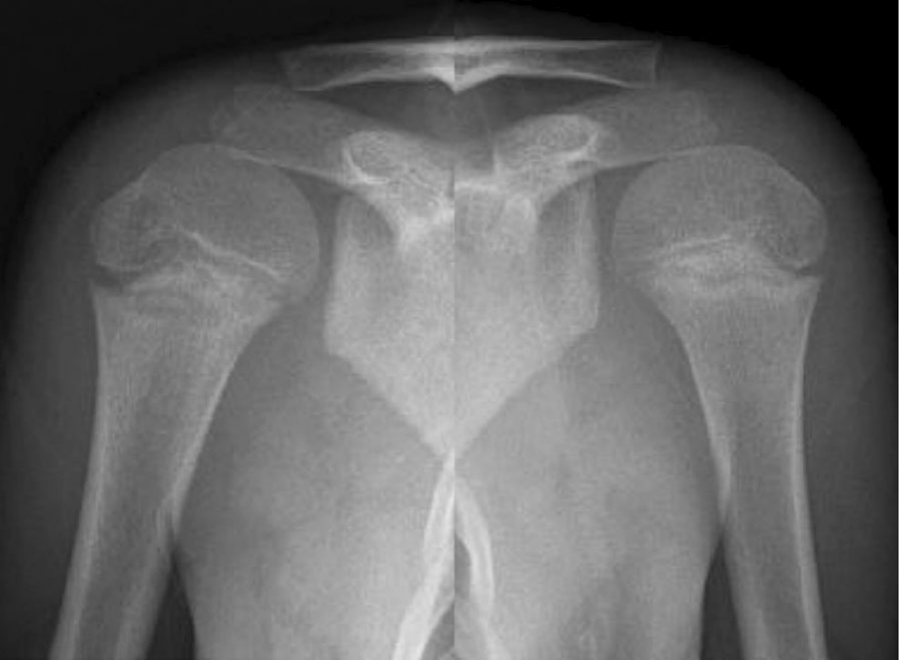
Little League shoulder. Anterior-posterior radiographs of bilateral proximal humeri of the same patient demonstrating widening of the proximal humeral physis on the right as compared with the normal left side. Reproduced from Stanley et al. [48], with permission
